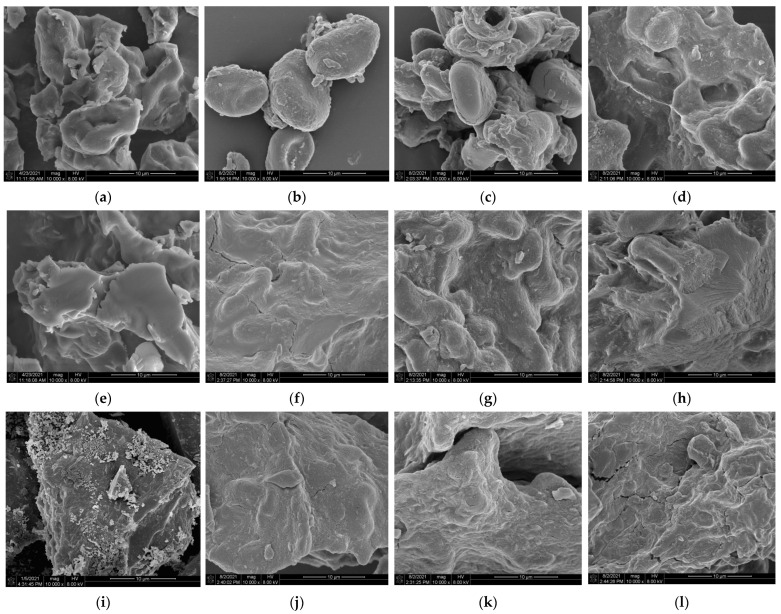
SEM images of LS-SNCs prepared using acid hydrolysis and U-LS-SNCs prepared using ultrasonically assisted acid hydrolysis. “W” and “d” represent ultrasonic power and acid hydrolysis days, respectively. U-LS-SNCs and LS-SNCs produced by employing (a) 0 W for 1 d, (b) 100 W for 1 d, (c) 150 W for 1 d, (d) 200 W for 1 d, (e) 0 W for 3 d, (f) 100 W for 3 d, (g) 150 W for 3 d, (h) 200 W for 3 d, (i) 0 W for 5 d, (j) 100 W for 5 d, (k) 150 W for 5 d, and (l) 200 W for 5 d.
